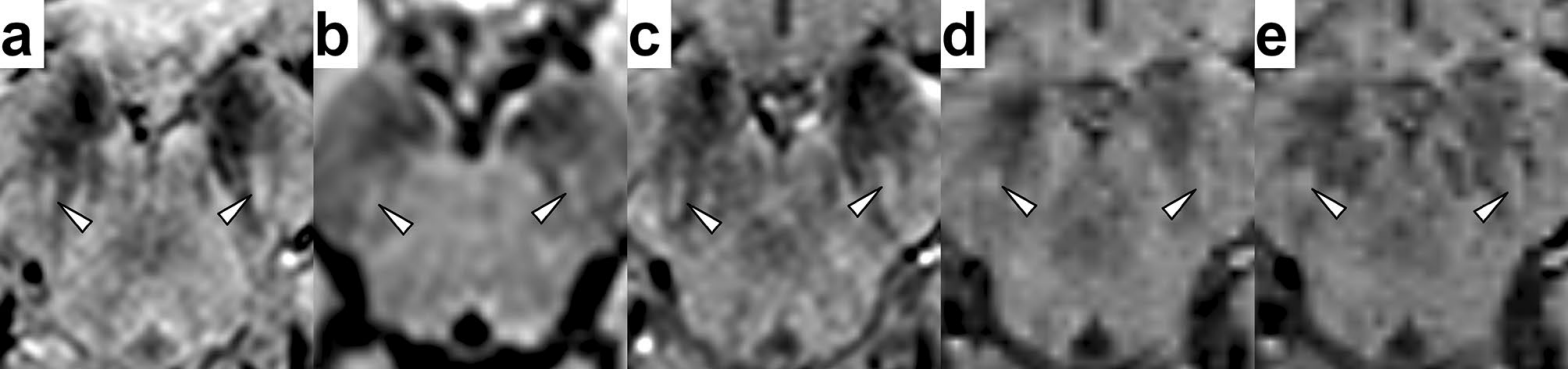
False-negative interpretation of the images of a 45-year-old woman with PD (Hoehn-Yahr stage: 1, MDS-UPDRS Part II: 2 points, MDS-UPDRS Part III: 4 points). Nigral hyperintensity was bilaterally rated as “clearly present” (white arrowheads) on EPISEG (a), FLAIR (b), MEDIC (c), MAG (d), and SWI (e) images as well. Images are shown in radiological convention (right = subject’s left) at a level below the red nucleus.
